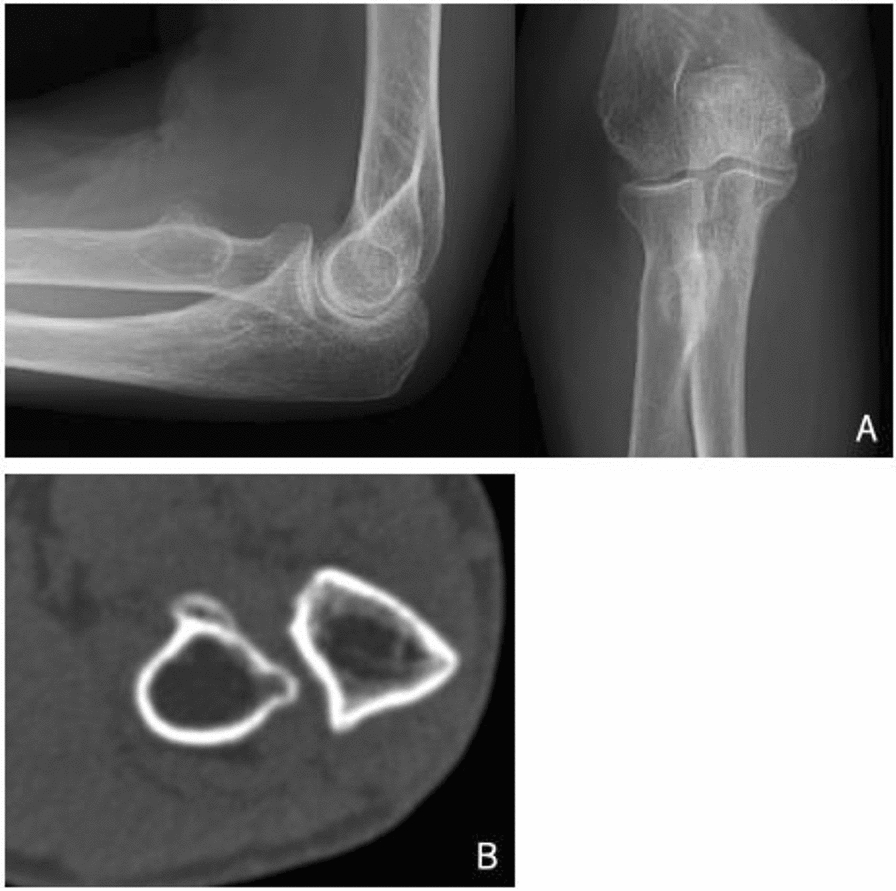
A Lateral and anteroposterior radiograph of the right elbow showed an abnormal prominence of the bicipital tuberosity of the radius. B Computed tomography of the right elbow showed abnormal spur formation of the bicipital tuberosity of the radius and the cortex of the ulna
